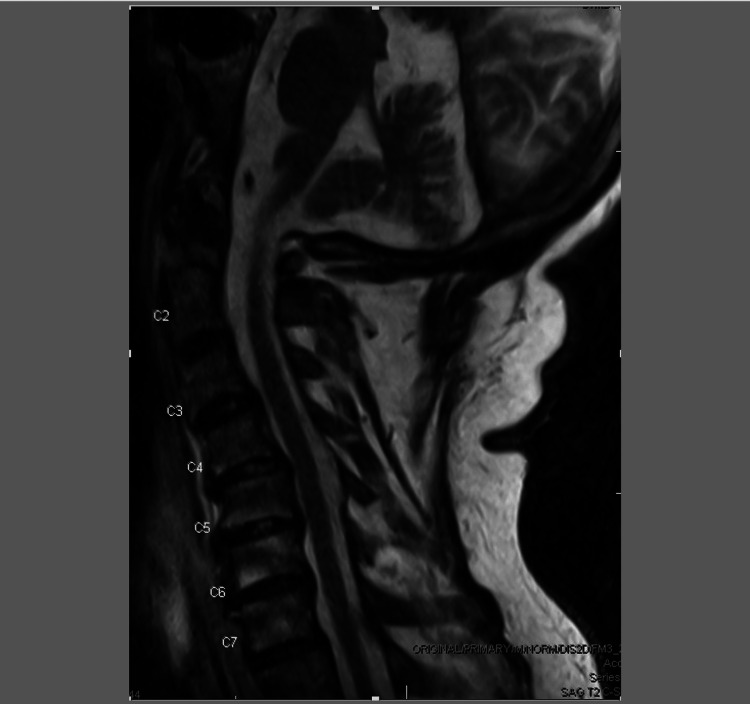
C-spine MRI T2 No abnormalities were noted on the patient's C-spine when imaged on an MRI.
